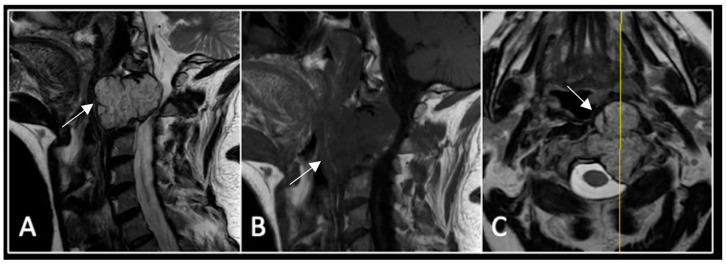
The MRI demonstrates a left-sided lobular mass (arrow) with invasion of the C2/3 vertebral body and epidural extension, but no cord compression. The yellow line on the axial image indicates the axial slice level. (A) T2W sagittal; (B) T1W sagittal; (C) T2W axial.
